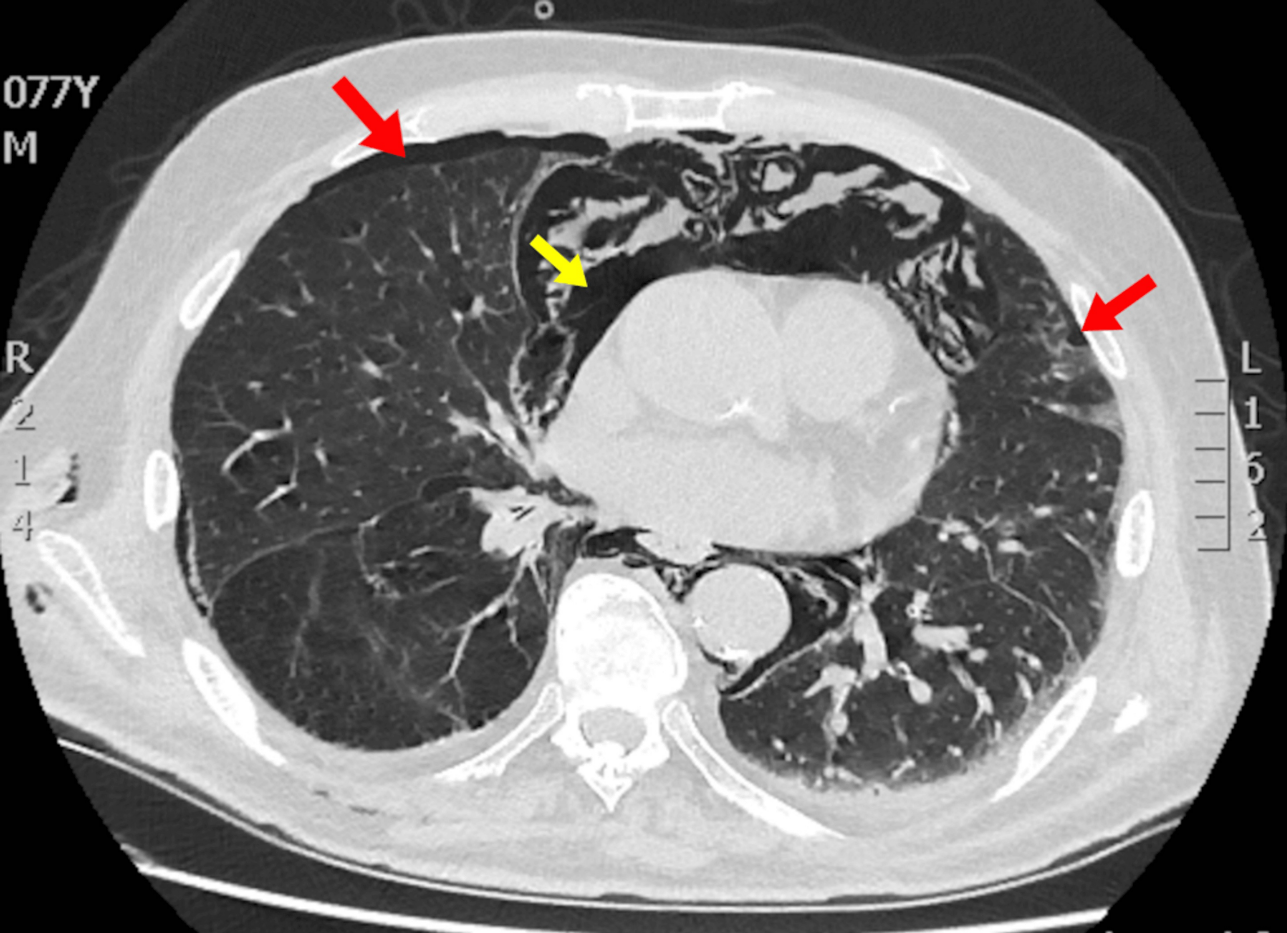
CT of the chest demonstrating pneumopericardium (yellow arrow) and small pneumothoraces (red arrows)
